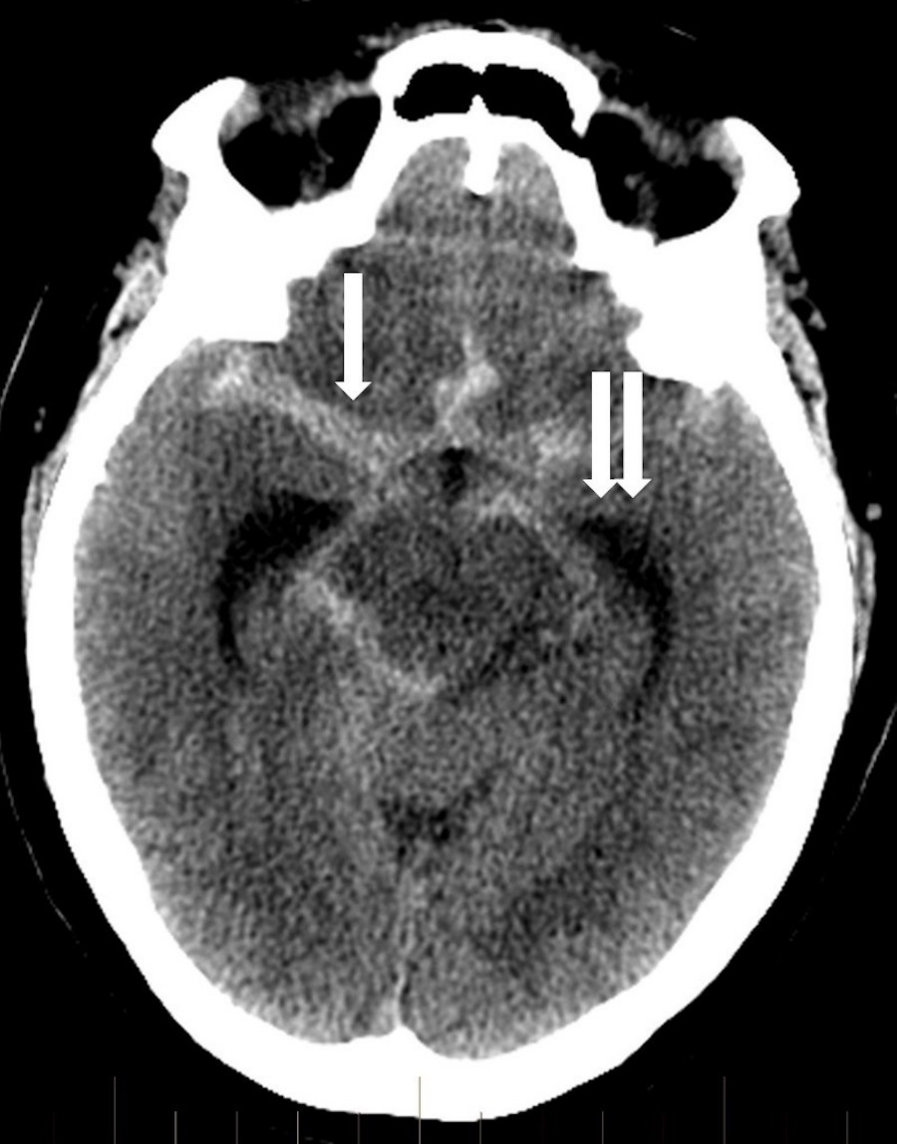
Non-contrast head computed tomography shows diffuse subarachnoid hemorrhage (arrow) and hydrocephalus (double arrow).
